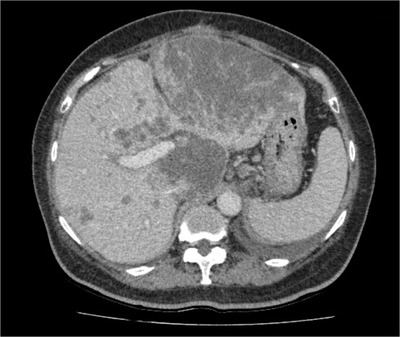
Six months after pylorus‐preserving pancreatoduodenectomy combined with extended lymph node dissection, the patient complained of abdominal pain and bloating. Computer tomography (CT) scans showed systemically metastasized to liver and peritoneum
